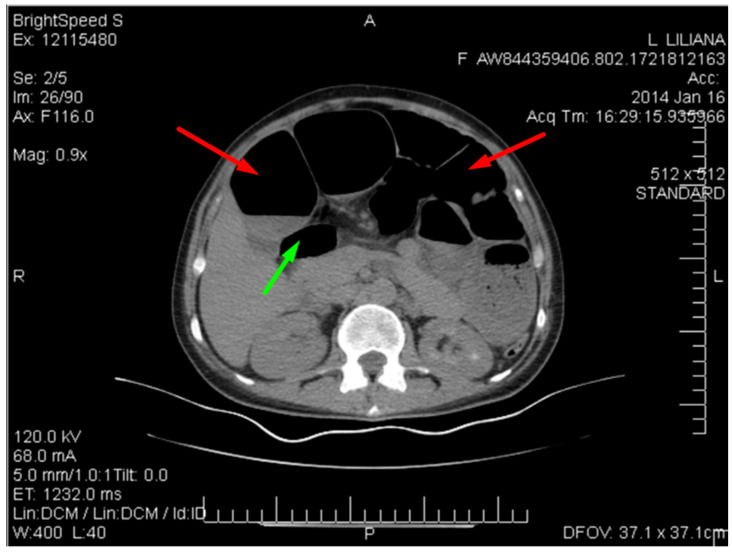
CT image of multiple dilated loops of small bowel with air (red arrows), and fluid (green arrow).
